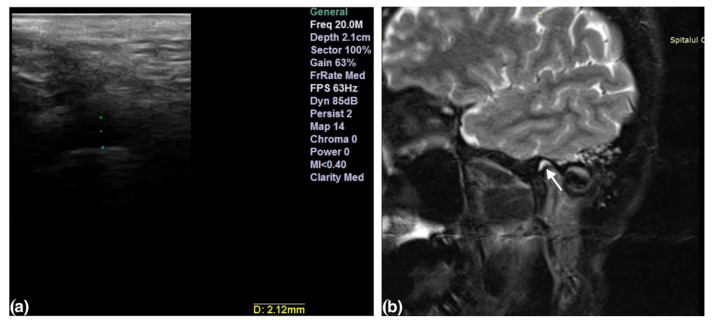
High-resolution 20 MHz US image (a) of joint effusion detected by direct visualization and abnormal capsular width (2.12 mm). Sagittal oblique T2-weighted TSE with fat suppression image (b) of the same joint with effusion (arrow) posterior to the disc in the closed-mouth position.
